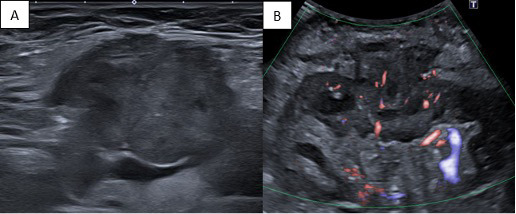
Ultrasound of malakoplakia in the transplanted kidney showing a hypoechoic (A), hypervascular (B) mass in the interpolar region.
